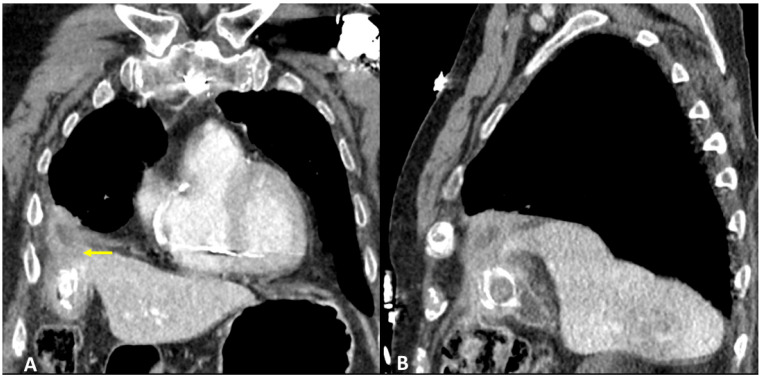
(A,B) Contrast-enhanced CT and coronal (A) and sagittal planes (B) show cholecystitis, edema, and thickening of pericholecystic adipose tissue, as well as an abscess at the gallbladder fundus adjacent to the right diaphragmatic pillar (arrow).
